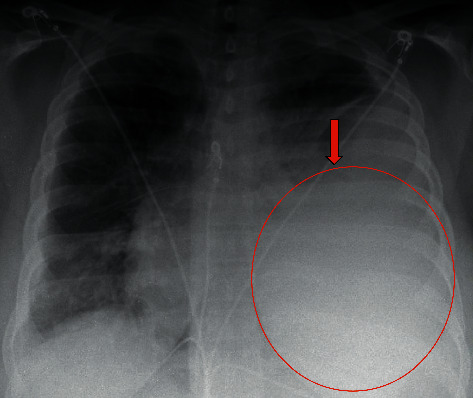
Chest x-ray portable revealing worsening left pneumonia with empyema.
